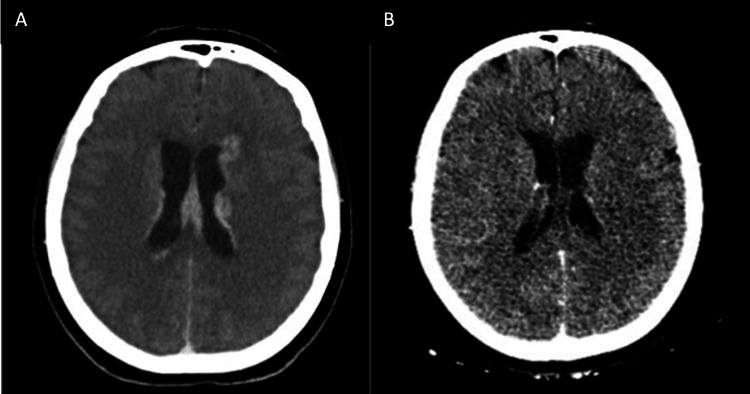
The computed tomography scan of the brain revealed periventricular enhancement and edema. B. Five months later, remission of periventricular enhancement and edema were noticed.
